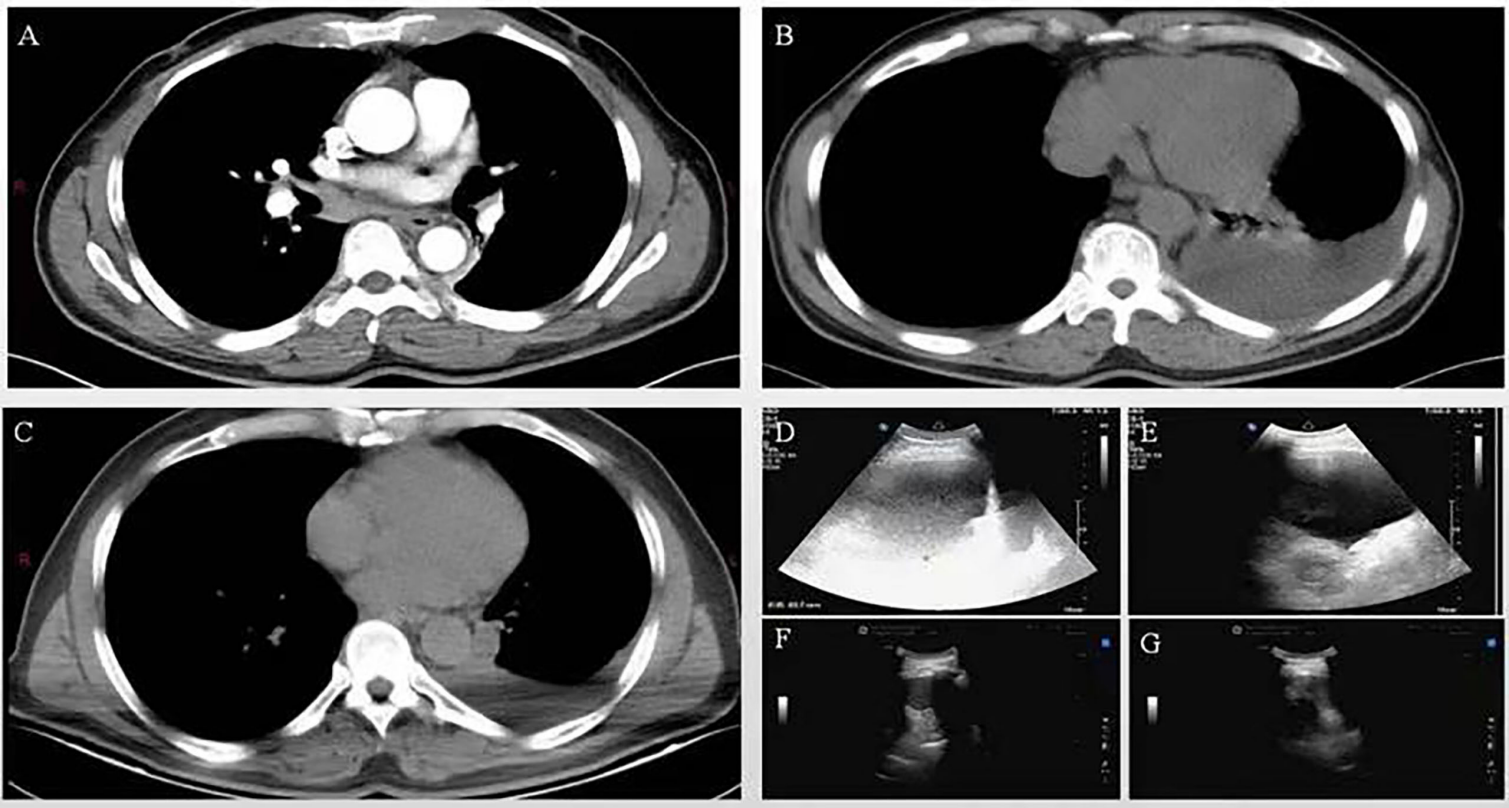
(A) In June 2021, CT suggested enlarged lymph nodes in the right hilar lung. (B, C) In February and March 2022, CT suggested a gradual decrease in pleural effusion. (D, E) In June 2021, a chest ultrasound suggested a large pleural effusion. (F, G) No pleural effusion was detected by chest ultrasound in April 2022.
